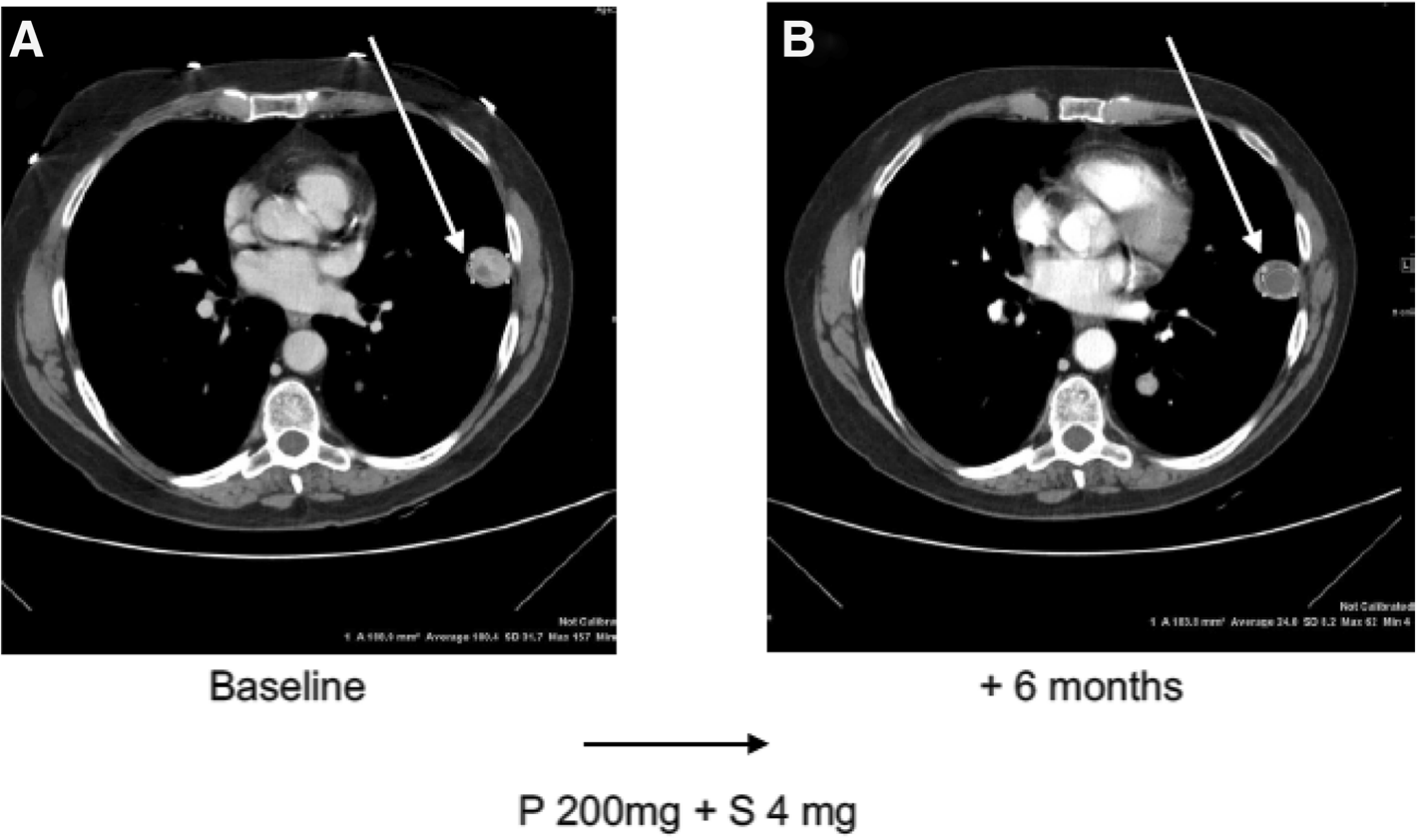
Response to P + S combination therapy. Computed tomography (CT) scan (arterial phase after contrast medium) of the chest. (a) Baseline (b) Six months after starting P + S combination therapy. Arrows indicate the response observed in the intrathoracic lesion, marked by decrease in tumor density
